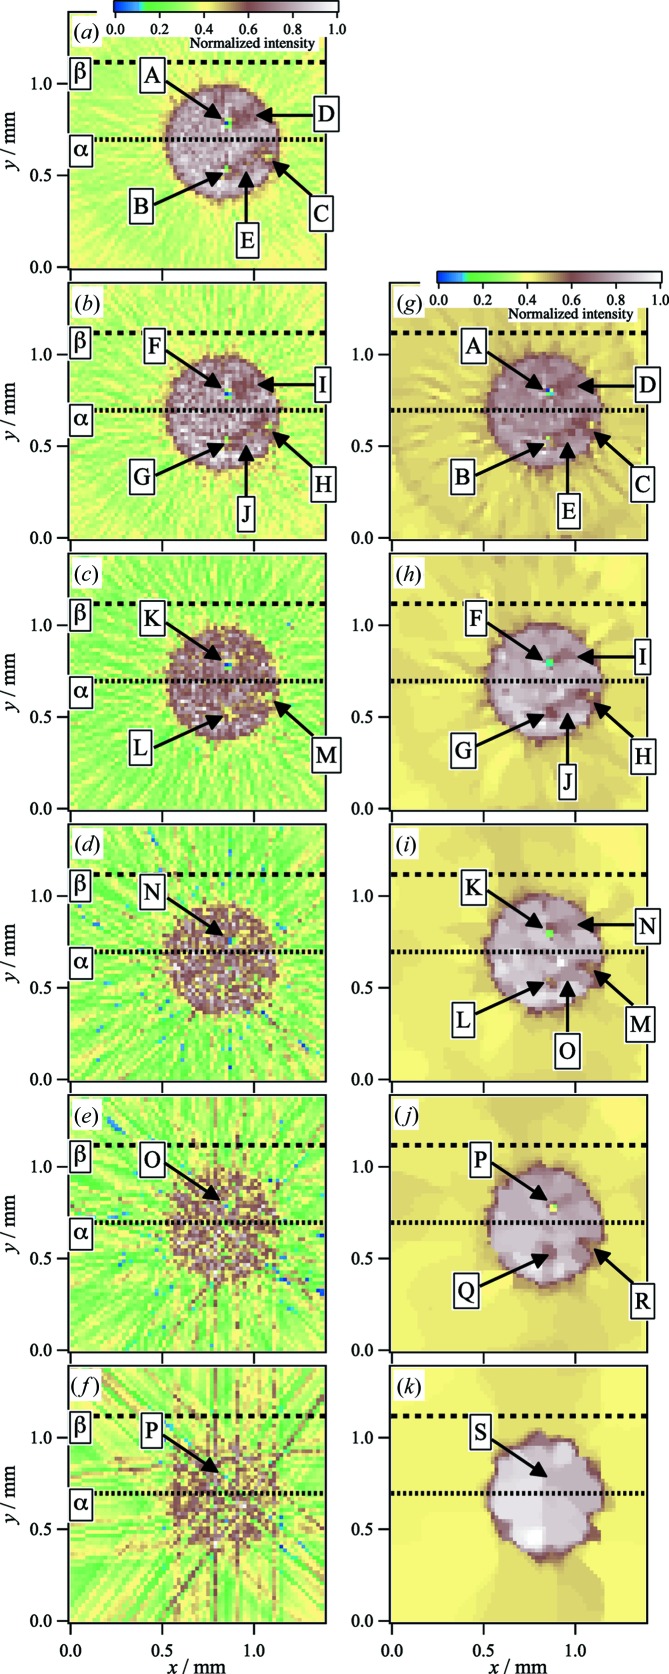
(Left) Reconstructed CT images as a function of Δθ. (*a*) Δθ = 1.0°; (*b*) Δθ = 3.0°; (*c*) Δθ = 6.0°; (*d*) Δθ = 12.0°; (*e*) Δθ = 24.0°; and (*f*) Δθ = 48.0°. The CT images were obtained from projections at the *q_y_* positions of 0.24 nm^−1^ in the in-plane profiles at *q_z_* = 0.80 nm^−1^. (Right) Recovered CT images using TV regularization for downsampling CT images: (*g*) Δθ = 3.0°; (*h*) Δθ = 6.0°; (*i*) Δθ = 12.0°; (*j*) Δθ = 24.0°; and (*k*) Δθ = 48.0°.
